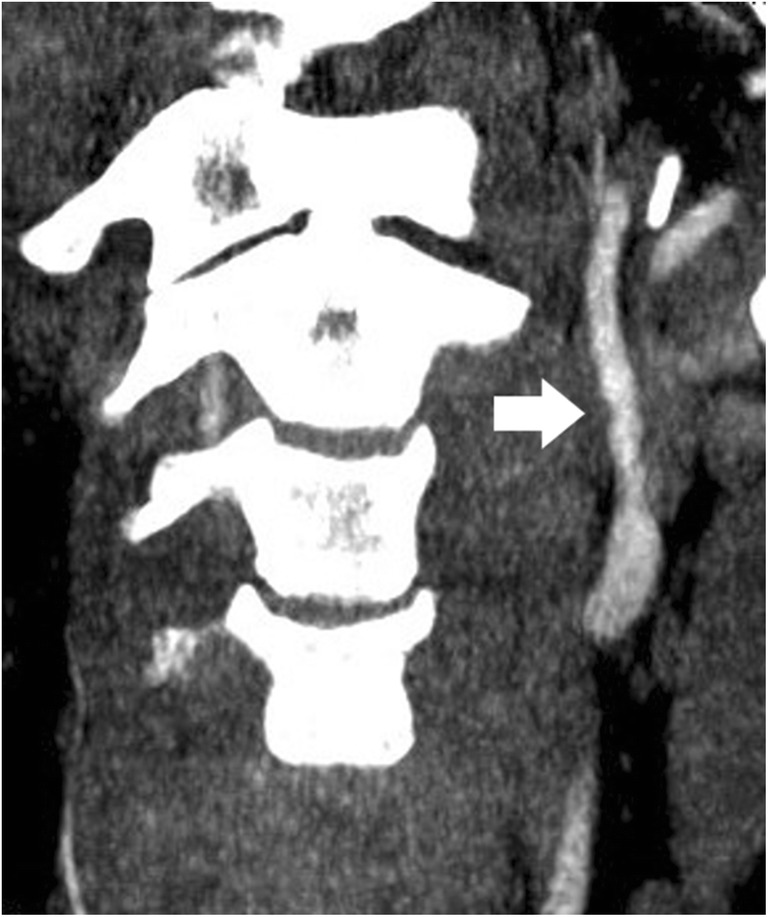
Grade I BCVI of the left ICA (arrow) in a 25-year-old female after MVA on CTA
